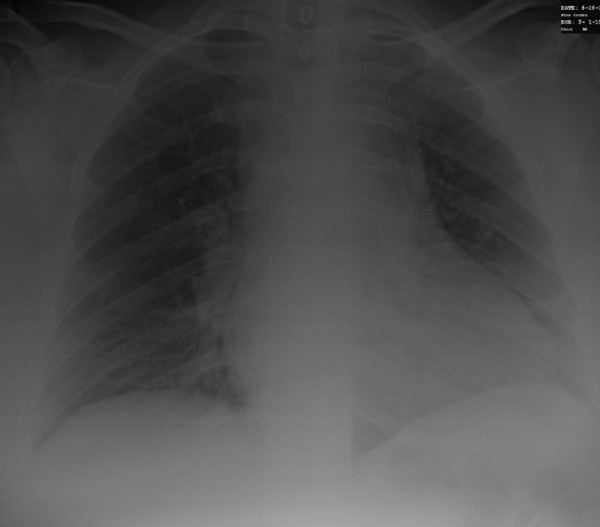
Chest radiograph showing left basilar scarring with blunting of the left costophrenic angle.
